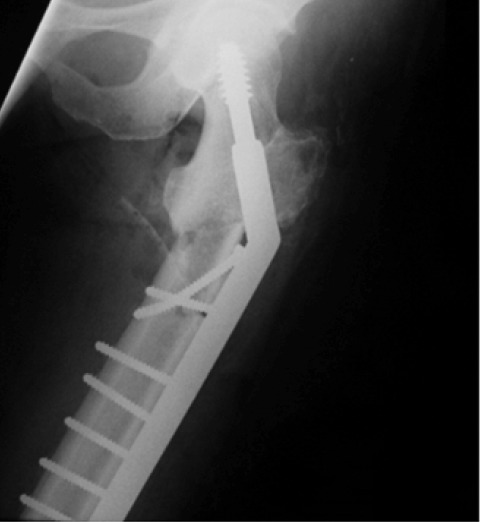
Radiograph showing dynamic hip screw fixation of the subtrochanteric fracture
